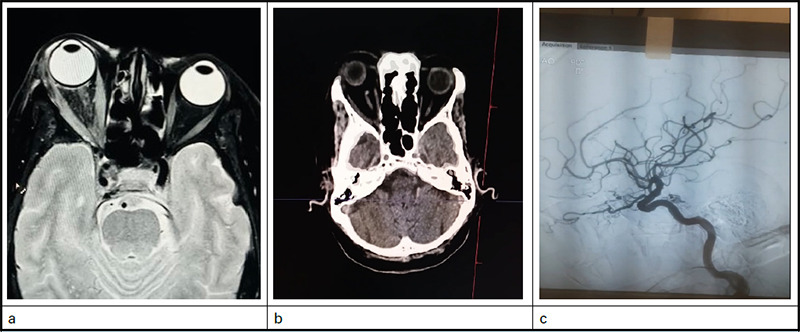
a. MRI showing Proptosis Right eye with extraocular muscle enlargement; b. CT scan showing prominent SOV; c. DSA of patient showing carotid cavernous fistulous connection between left ICA to right cavernous sinus.
